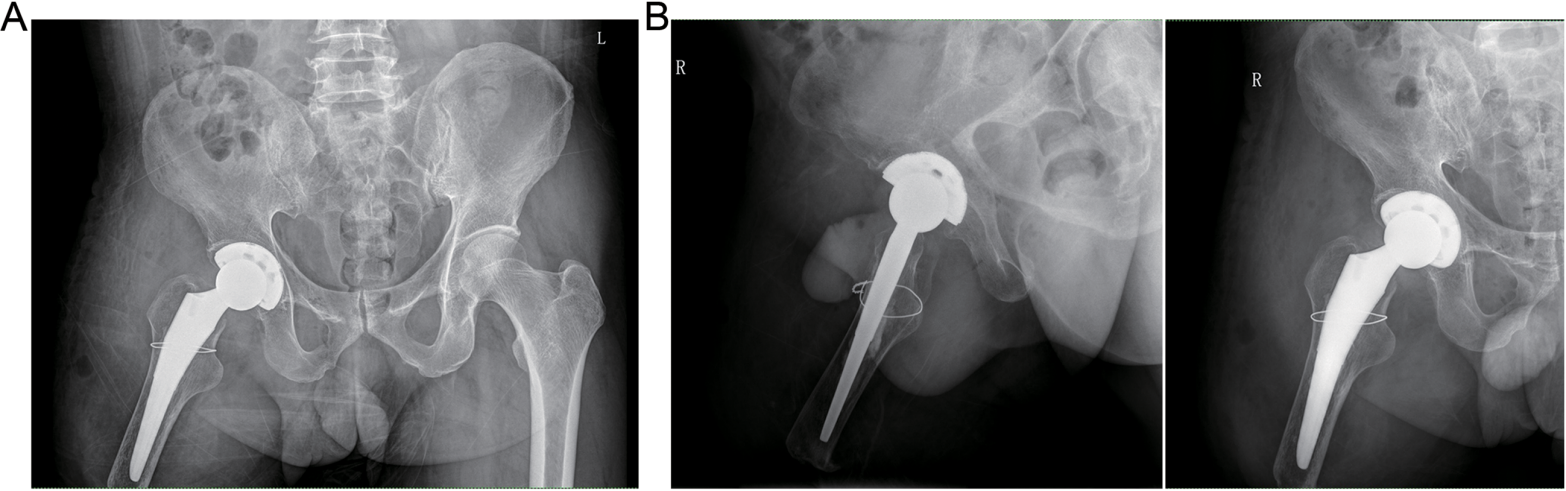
Postoperative radiograph of the patient at 1 day. (A) Anteroposterior pelvic x-ray. (B) Anteroposterior x-ray of the right hip joint and lateral x-ray of the right femoral neck.
